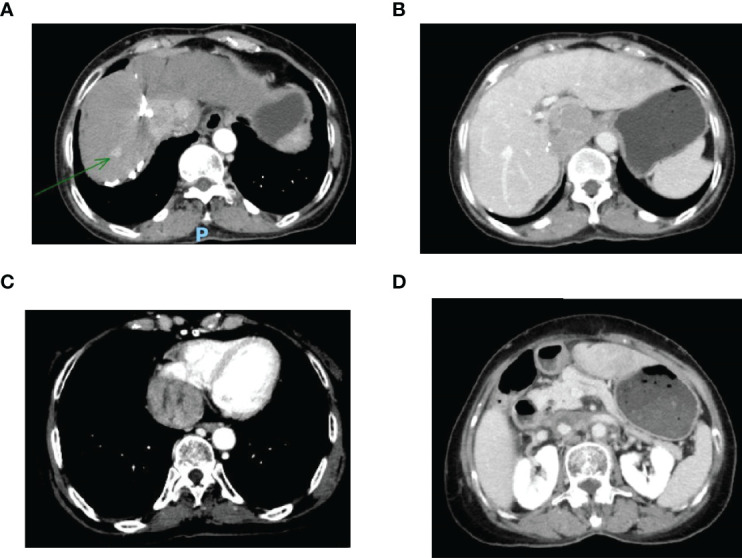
(A) Enhanced CT revealed a mass tumor in the S5/S8 segment of the liver; (B) The tumor thrombus in the inferior vena cava; (C) The tumor thrombus in the right atrium; (D) The tumor thrombus in the left renal vein. CT, computed tomography.
